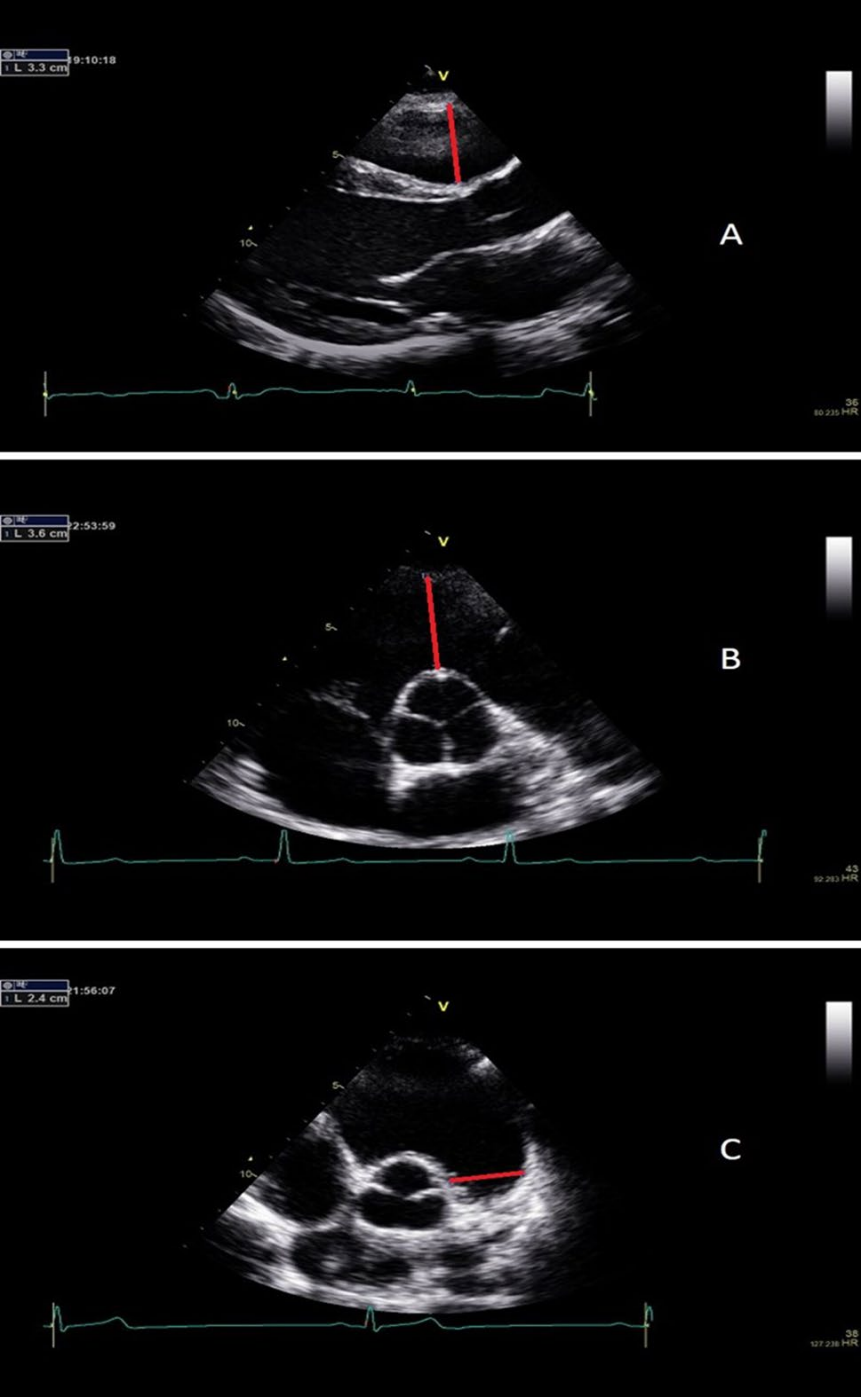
Parasternal views demonstrating RV outflow dimensions. a (Proximal) = RVOTplax; (b) (Proximal) = RVOT1; (c) (Distal) = RVOT2, Exemplar dimensions
